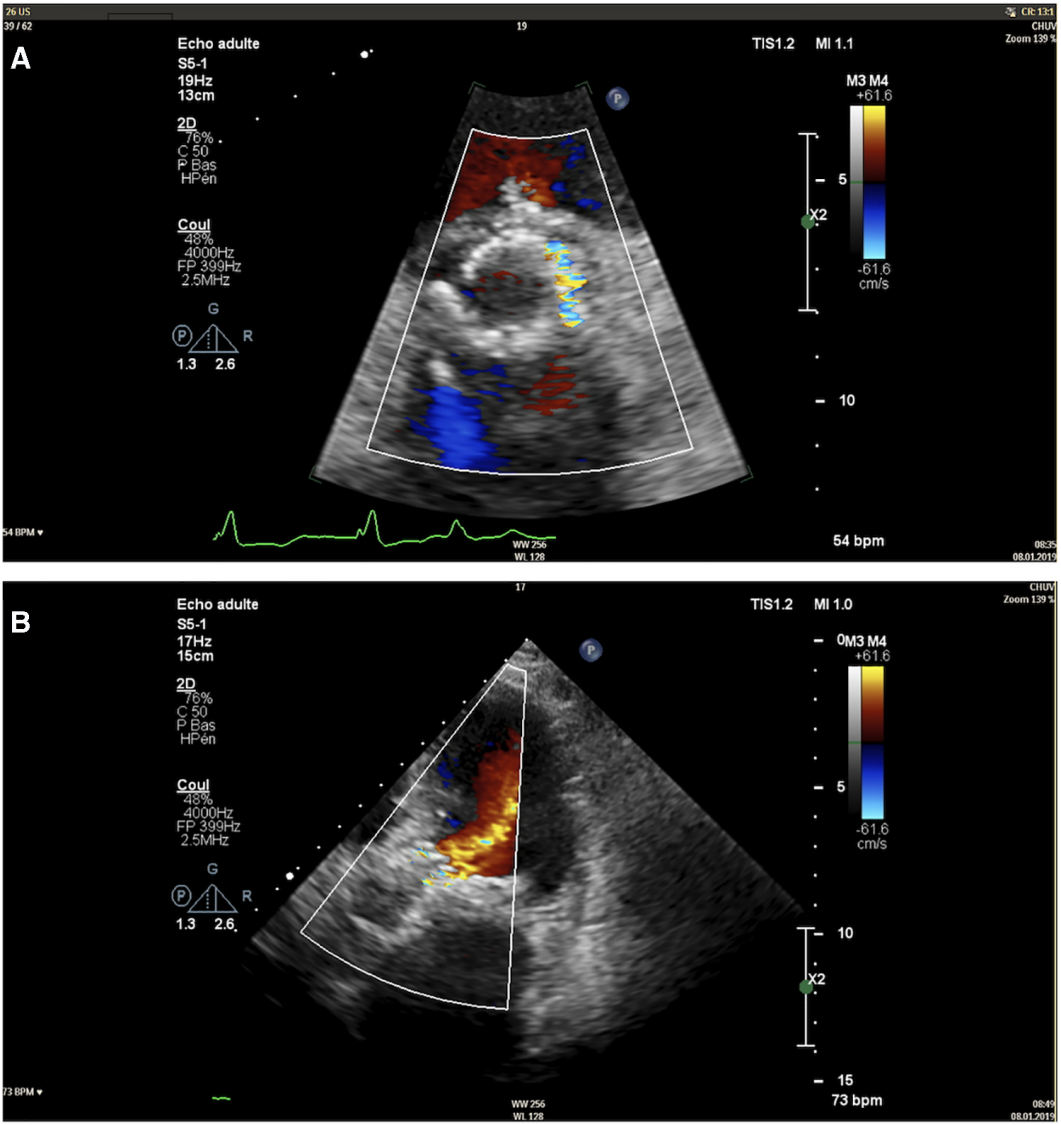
Transthoracic echocardiography image of the paravalvular leak in short-axis view (A) and apical five-chamber view (B).
